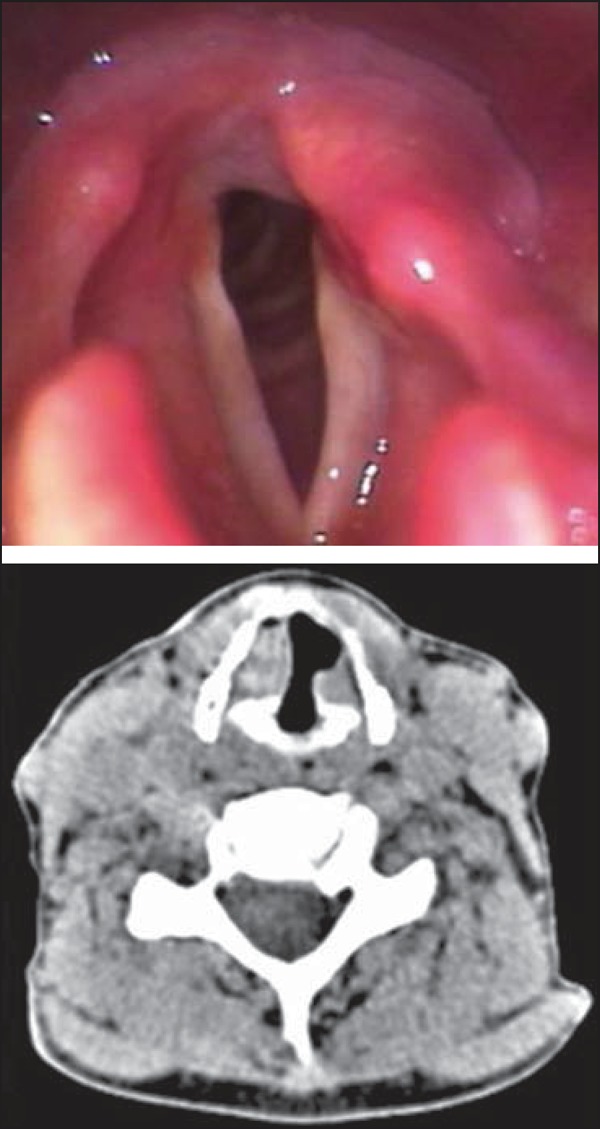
Documentation of videolaryngoscopy and axial CT section (soft tissue window)
